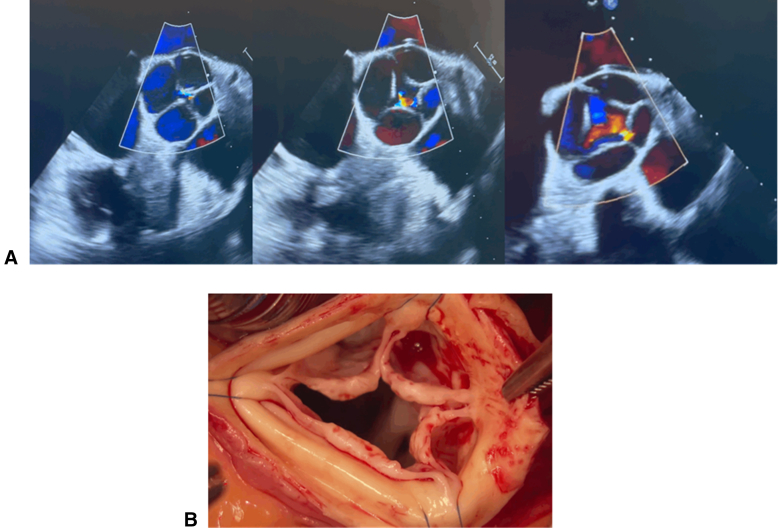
Preoperative transthoracic echocardiography (A) and surgical (B) view.
